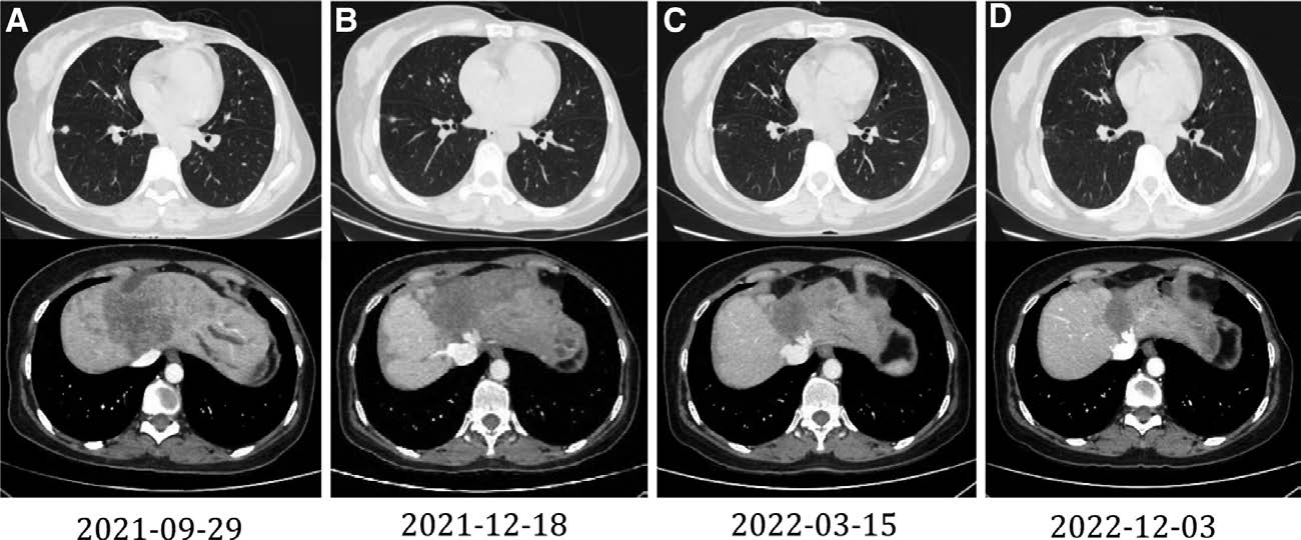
Changes in lung and liver lesions on CT scan. (A) Before treatment (2021-09-29), (B) After 2 months of treatment (2021-12-18), (C) After 5 months of treatment (2022-03-15), (D) After 14 months of treatment (2022-12-03). CT = computed tomography.
